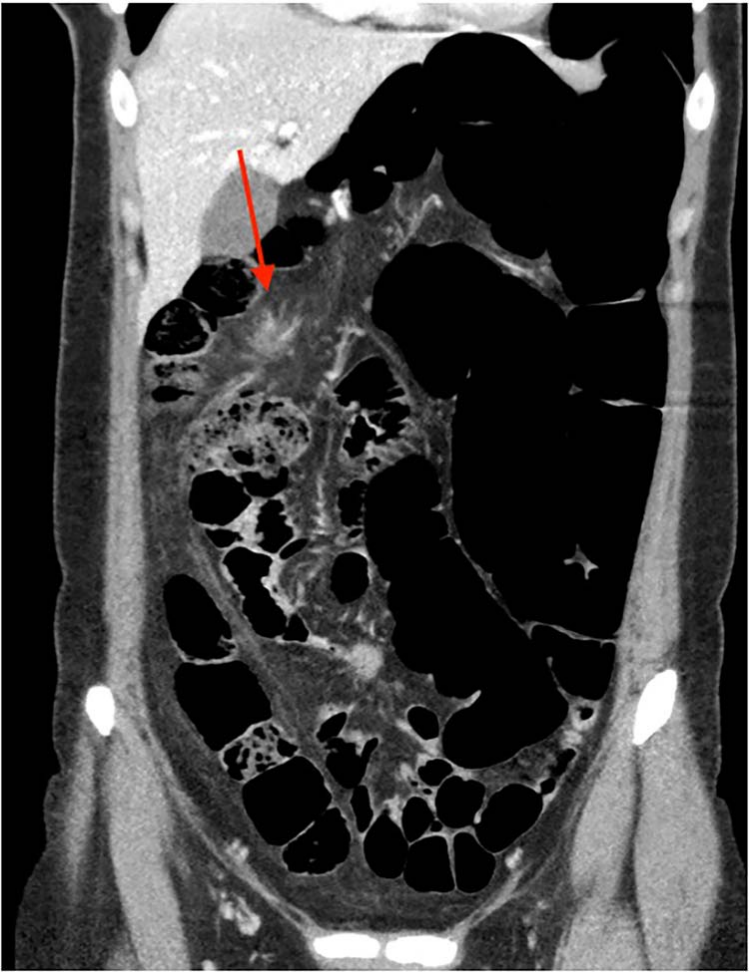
CT abdomen pelvis in 2019 (coronal).
